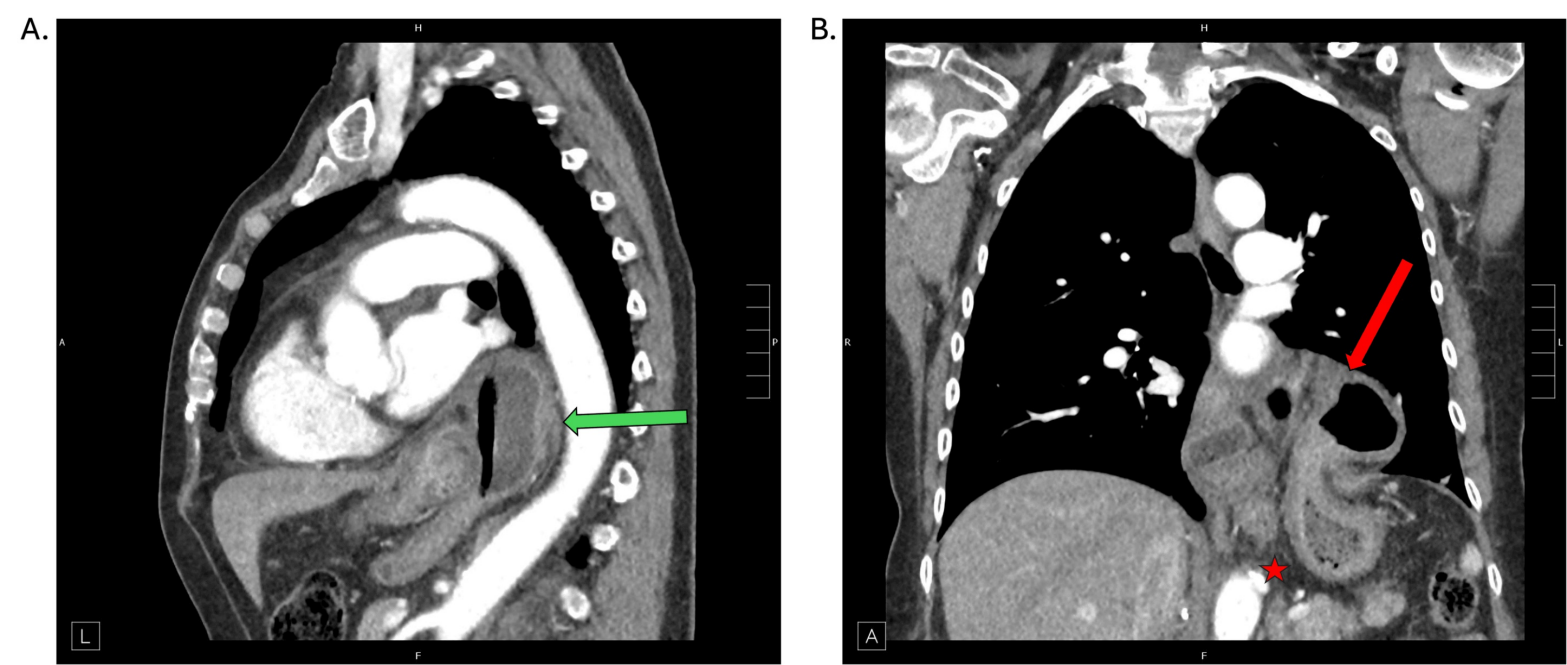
Thoracoabdominal CT scan Thoracoabdominal CT scan with sagittal (A) and coronal (B) views. Hernia sac above the diaphragm is seen in sagittal view (green arrow). The gastric fundus (red arrow) is superior to the preserved gastroesophageal junction (red star) CT: computed tomography
